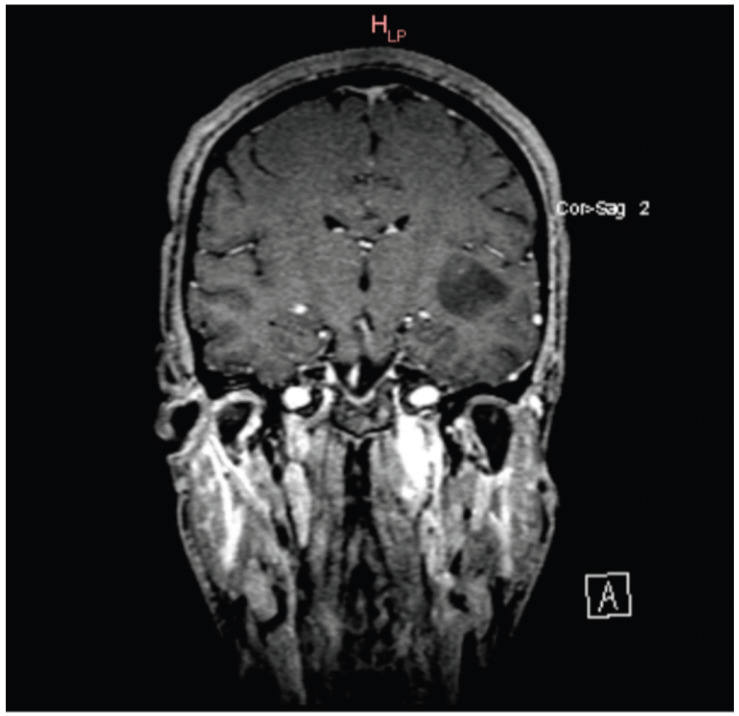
Brain MRI of a left insular–temporal lesion in coronal section.
